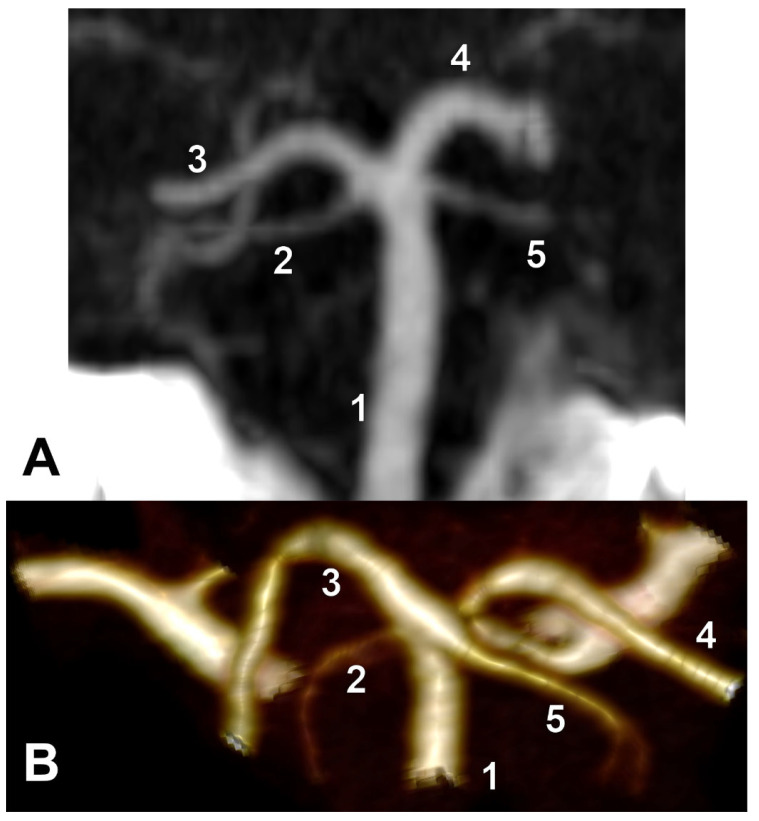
Types F of the bilateral combination of superior cerebellar arteries origins. CT angiograms. (A) Normal posterior cerebral arteries, coronal slice, anterior view. 1. BA; 2. right SCA; 3. right PCA; 4. left PCA; 5. left SCA. (B) Fetal type of the right posterior cerebral artery, three-dimensional volume rendering, posterior view. 1. BA; 2. left SCA; 3. left PCA; 4. right fPCA; 5. right SCA.
